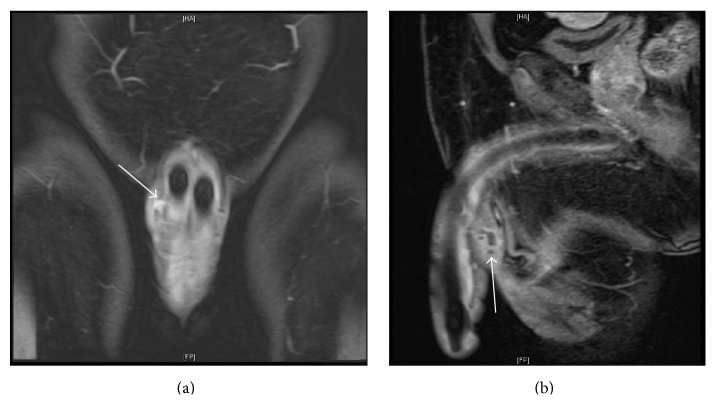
Coronal (a) and sagittal (b) MRI views of the collection revealing its multiloculated structure and communication with the right corporal body (arrows).
